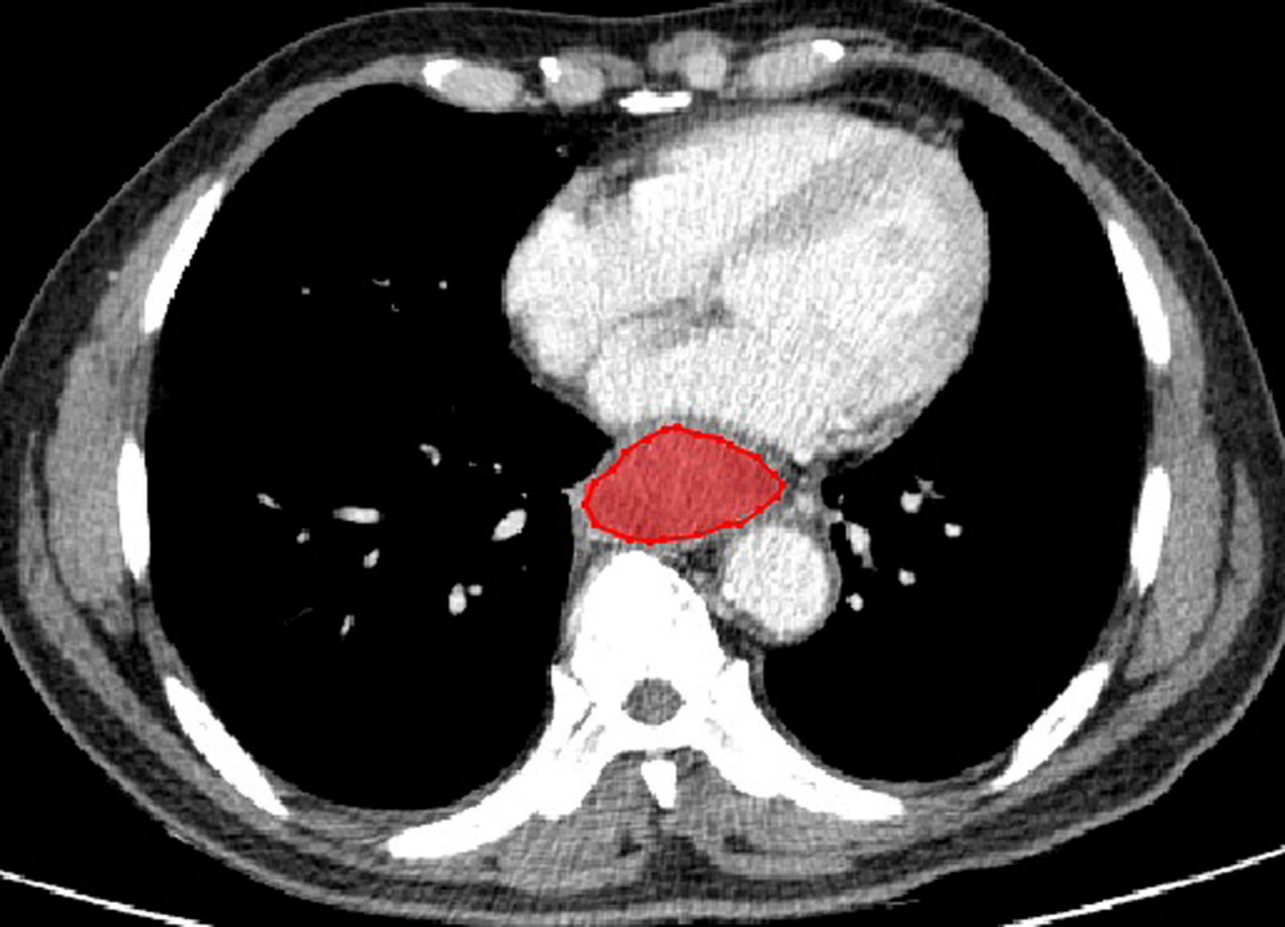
A enhanced CT of a 69-year-old man with esophageal NEC in the middle segment of esophagus (cT3N2M0). There is a moderately enhanced lesion with well-defined margin without necrosis and calcification. The red outline depicts an example of the drawn volume of interest (VOI) covering the largest image slice of the primary lesion.
